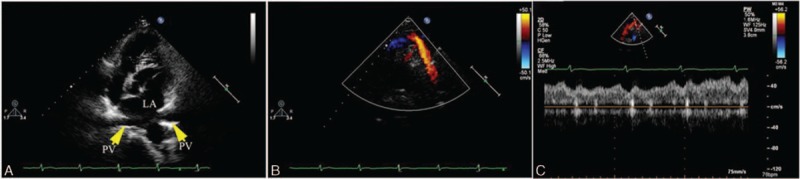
A case misdiagnosed as supracardiac partial anomalous pulmonary venous connection. (A) Two openings of the pulmonary vein into the left atrium can be detected clearly by apical view. (B) An upward venous drainage to left innominate vein as shown via suprasternal view, which was identified as anomalous azygos vein by computerized tomography angiography. (C) Pulse Doppler of this anomalous vein showing low velocity and nonphasic flow.
